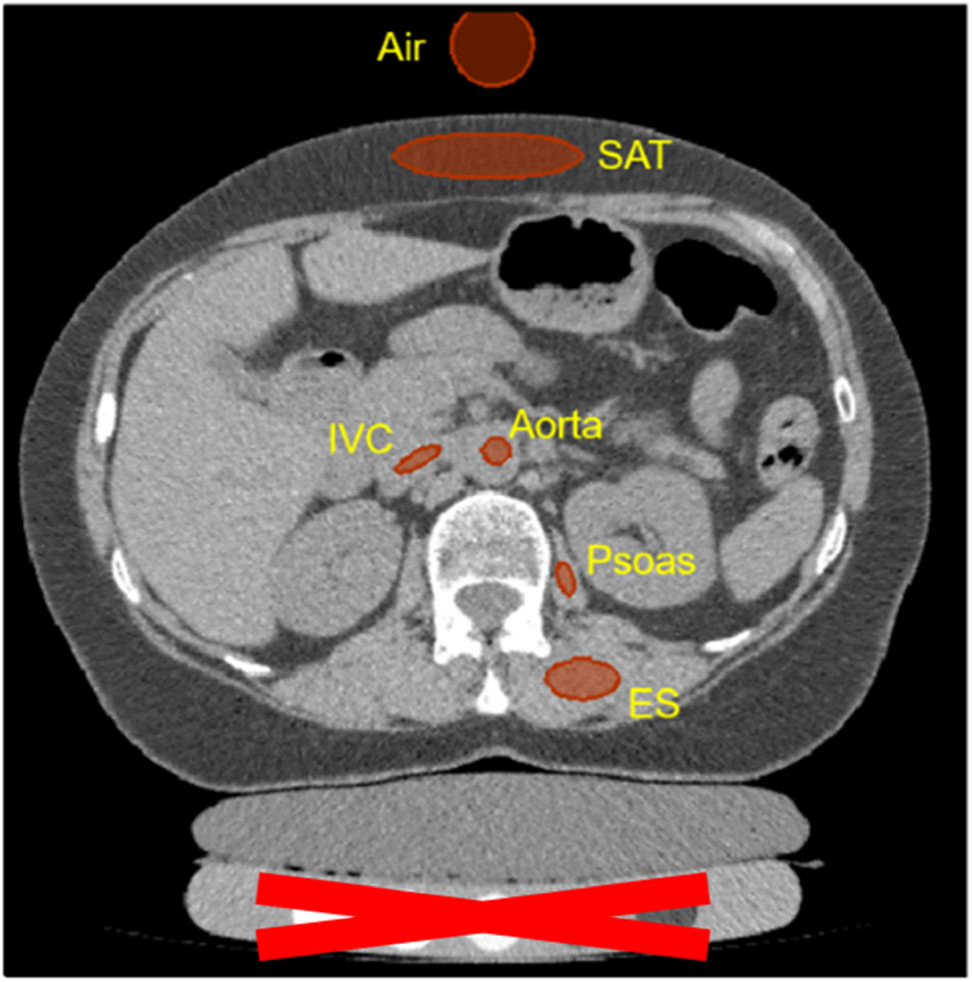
Volumes of interest used to determine CT values of tissues used for internal calibration: muscle of the erector spinae (ES) and the psoas muscle, blood in the aorta and the inferior vena cava (IVC), and subcutaneous adipose tissue (SAT)
